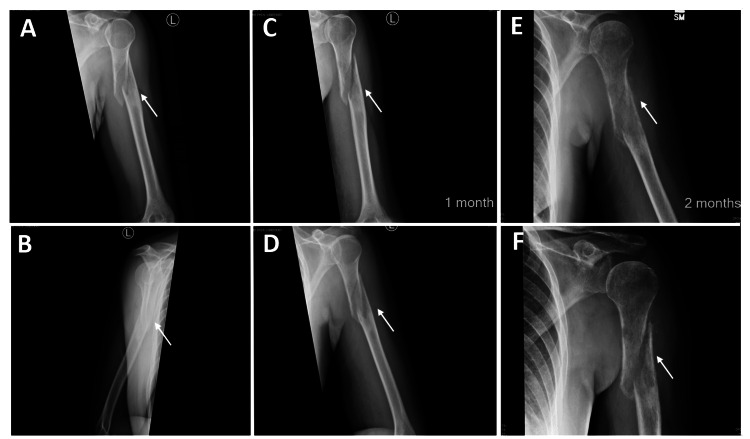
Series of radiographs explicit the progression of pathological fracture at the proximal third of the humeral shaft A, B: Initially frontal and later radiographs showed an acute oblique fracture of the proximal diaphysis of the left humerus with approximate half shaft width and lateral displacement of the distal fracture fragment. C, D: One-month follow-up radiograph again showed a mild interval improvement of alignment of the fragments. The distal component remains ununited and displaced laterally. E, F: Two-month follow-up radiograph showed unaltered alignment and positioning with ongoing mild impaction and apex medial/anterior angulation. No Significant interval callous formation. The union is not complete. There appears to be a moth-eaten pattern of the adjacent bony matrix concerning pathological fracture.
